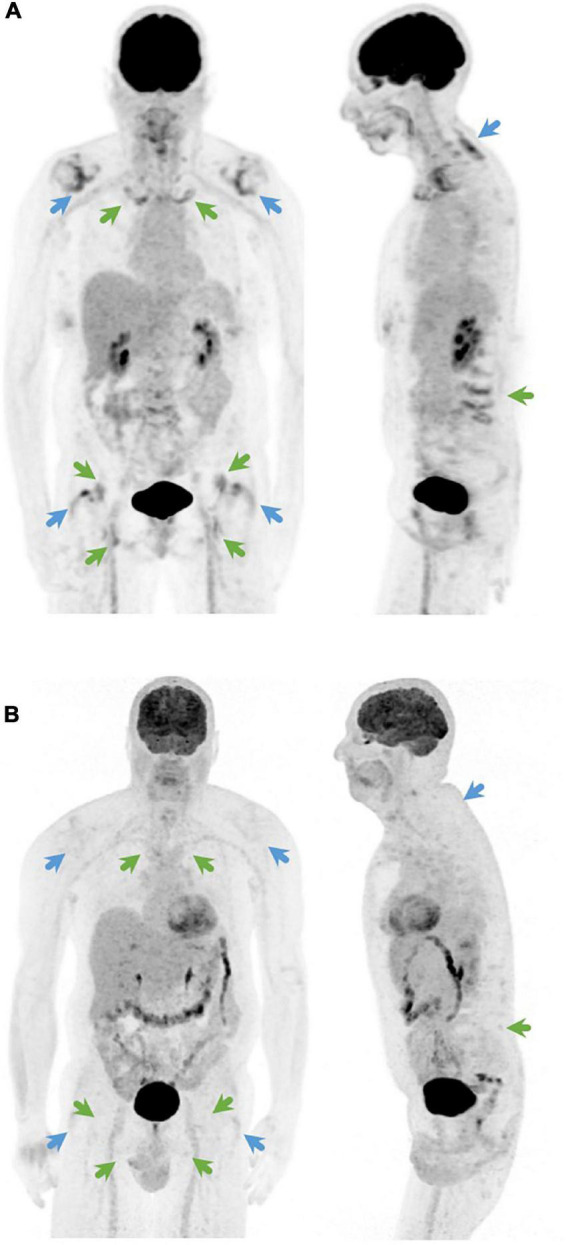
Coronal (left) and sagittal (right) ^18^F-FDG-PET image of (A) a PMR patient with a Leuven score of 24 and a Leuven/Groningen score of 14 and (B) a patient with fibromyalgia as final diagnosis with a Leuven score of 3 and a Leuven/Groningen score of 1. The Leuven score is the sum of joints indicated by green and blue arrows. The Leuven/Groningen score is the sum of joints indicated only by green arrows.
